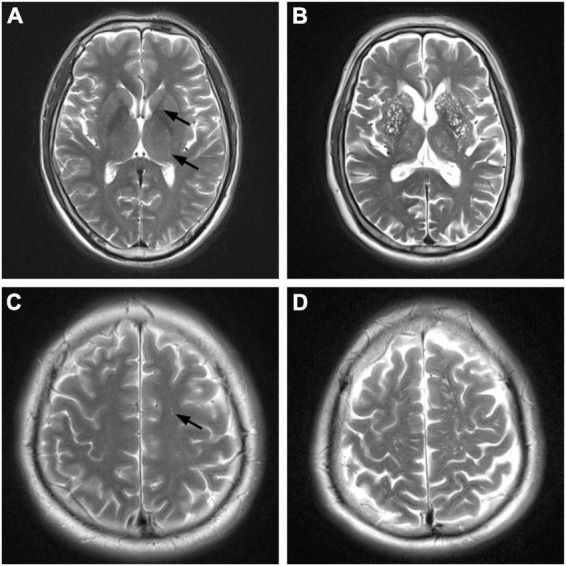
Magnetic resonance imaging (MRI) manifestations of different degrees of enlarged perivascular space (EPVS) (T2-weighted imaging). BG, basl ganglia; CS, centrum semiovale. (A) Mild BG-EPVS. (B) Severe BG-EPVS. (C) Mild CS-EPVS. (D) Severe CS-EPVS.
